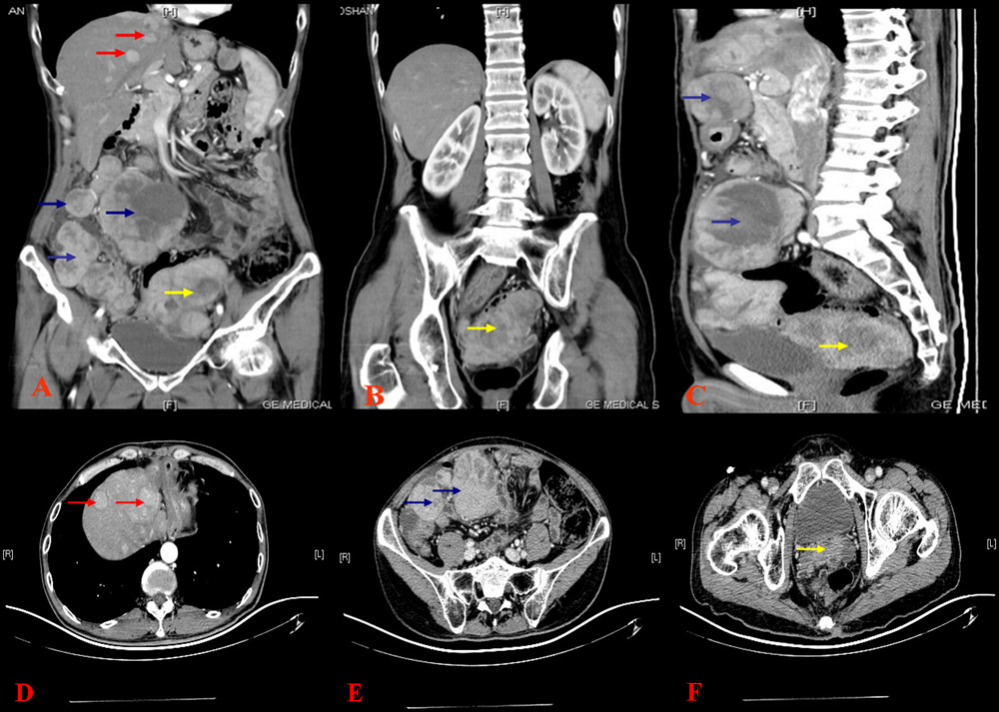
Computed tomography (CT) revealed multiple intrahepatic recurrent or metastatic foci and metastatic foci in abdominal and pelvic cavity. Red arrow, intrahepatic recurrent or metastatic foci; blue arrow, metastatic foci in abdominal; yellow arrow; metastatic foci in the seminal vesicle region.
